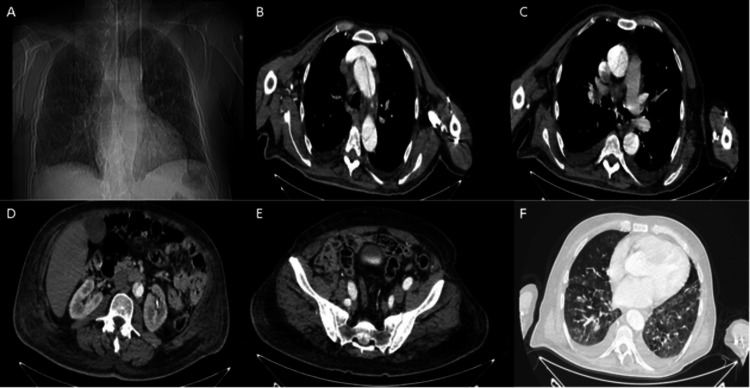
(A) Current chest radiogram, (B, C) current axial contrast-enhanced thoracoabdominal CT images of the patient, (D, E) a dissection line progressing to the iliac bifurcation, (F) axial thorax tomography images of the patient with sequela images of lung involvement areas due to COVID-19 infection CT: computed tomography; COVID-19: coronavirus disease 2019
